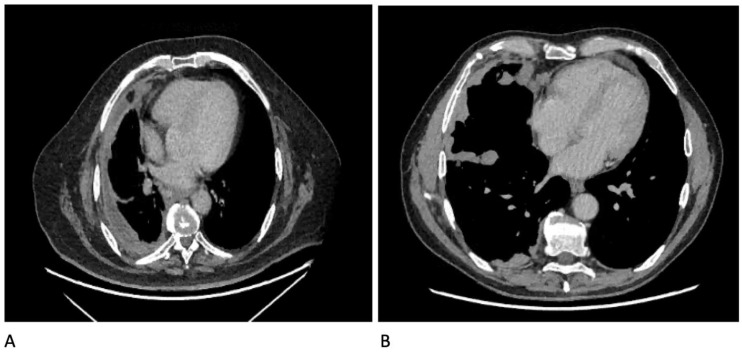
CT images showing some features of malignant pleural disease: (A) Circumferential pleural thickening; (B) Nodular pleural thickening involving mediastinal surfaces and fissures.
